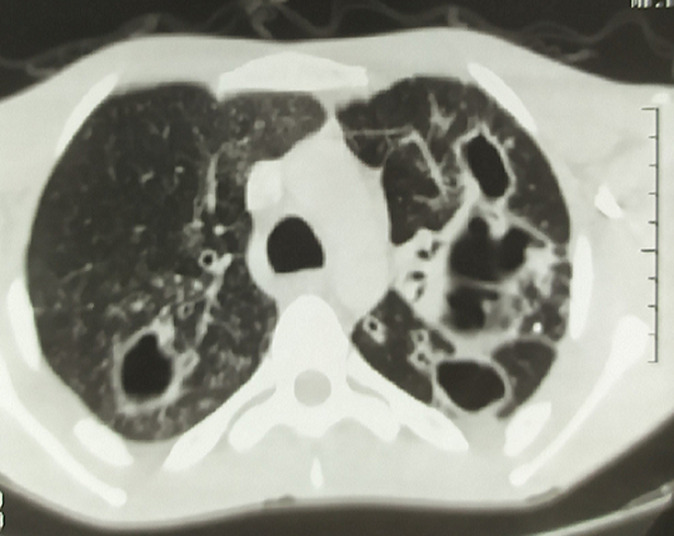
chest CT scan: excavated lesions of the pulmonary apex very suggestive of tuberculosis
